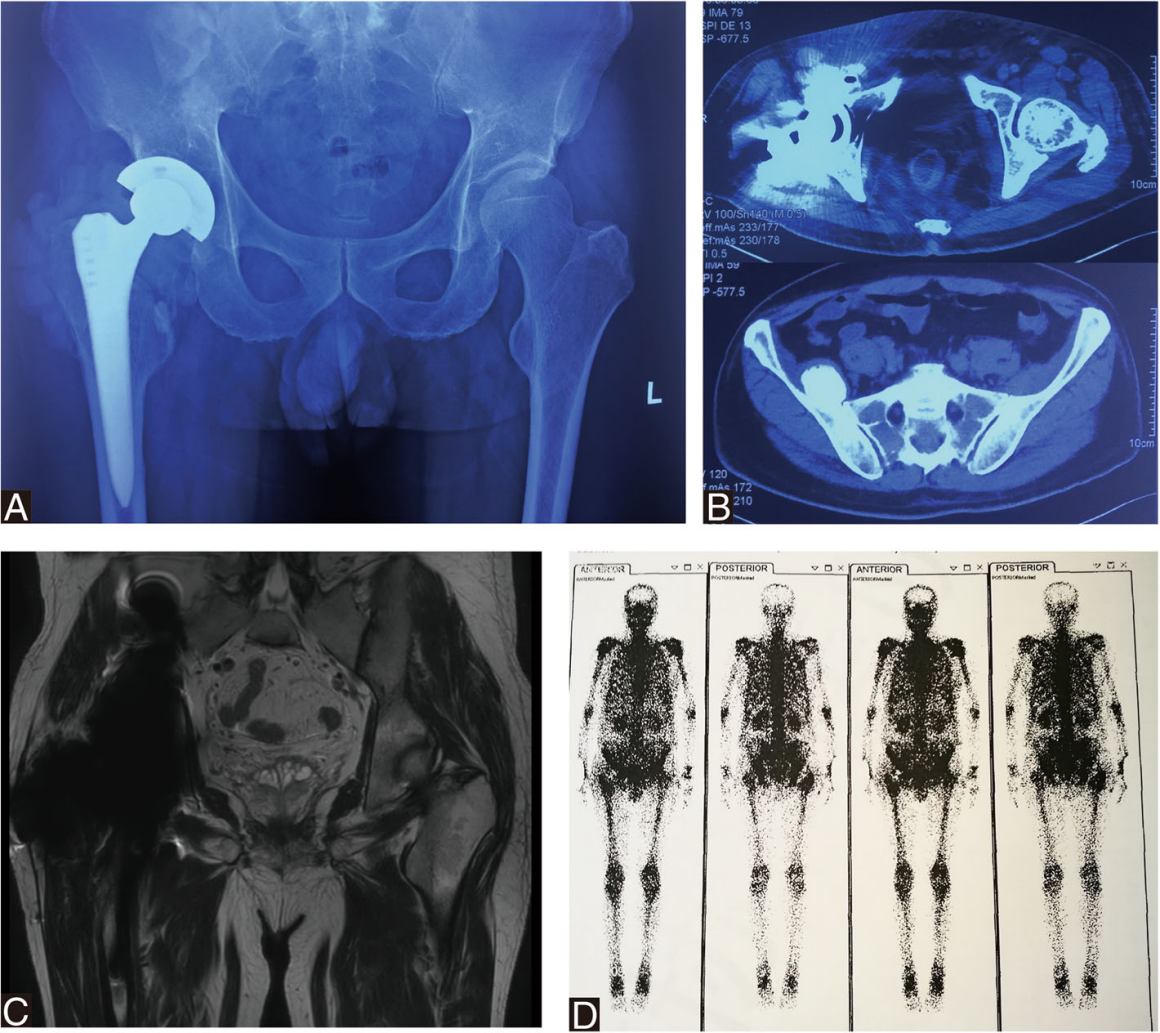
Pseudotumor formation after revision MoP Total hip arthroplasty. a Anteroposterior radiograph showing extensive soft tissue mass around the right hip prosthesis and a mass in pelvis. b A transaxial view of the CT showed extensive right hip high density shadow and a high density mass in pelvis. c MRI T2image showed significant artifact in the region of the right hip MoM prosthesis. Surrounding the prosthesis was extensive soft tissue and muscle edema affecting the gluteus muscles, piriformis, rectus femoris, obturator externus, pectineus, and the adductor bundle. d
